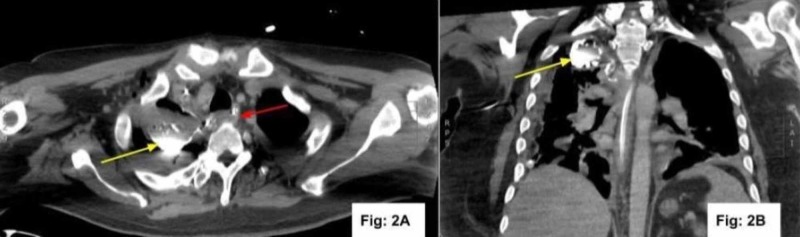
CT of the chest after incision and drainage of the abscess CT with contrast axial (Fig: 2A) and coronal (Fig: 2B) reconstruction demonstrates orally administered barium contrast in the right apical pleural space (yellow arrow) and decrease in size of intramural esophageal abscess (red arrow) consistent with esophageal pleural fistula CT: computed tomography
